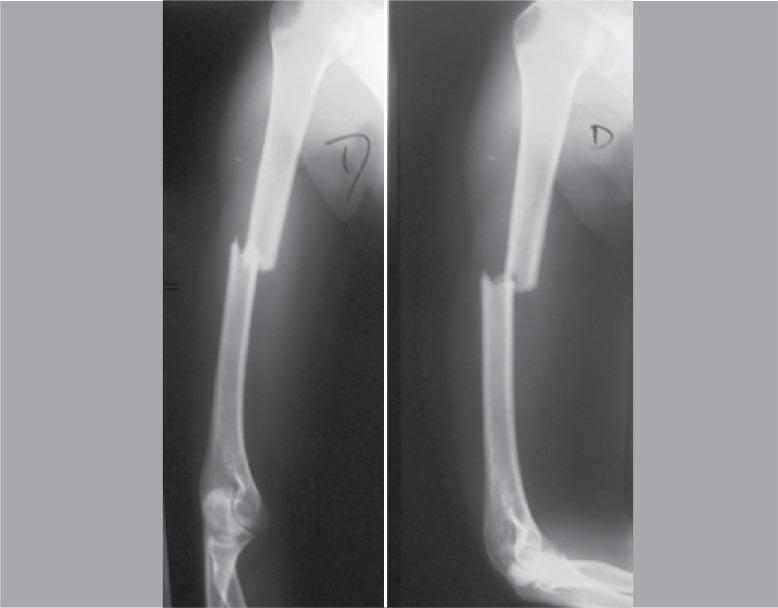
Preoperative anteroposterior and lateral radiographs incidence.
